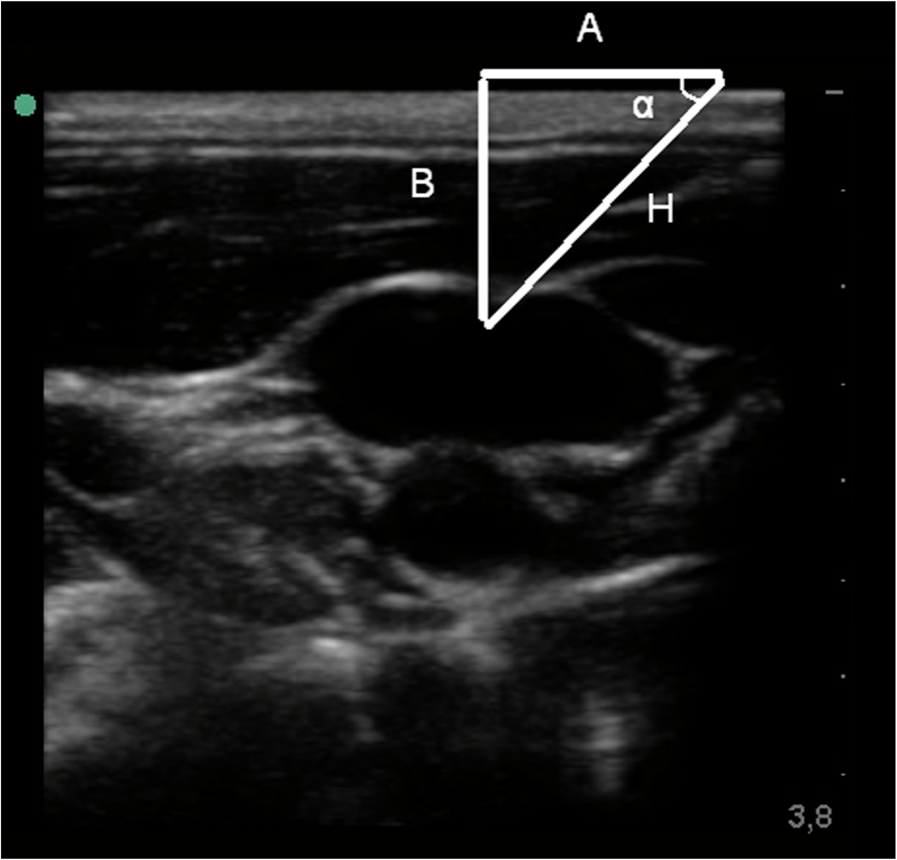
Internal jugular vein ultrasound anatomy. *H* hypotenuse; A and B are catheti of the triangle
